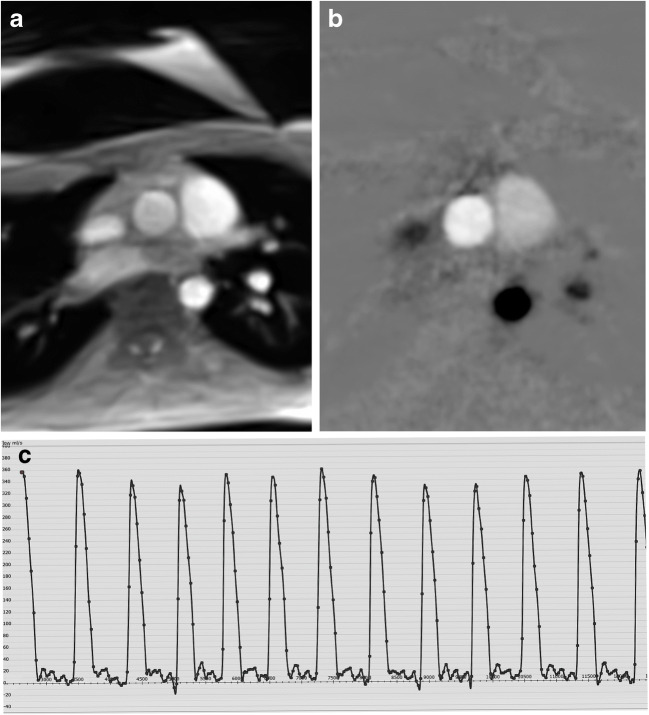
Real-time phase-contrast flow MRI of the ascending aorta in a 17-year-old male adolescent with funnel chest and vacuum bell at low pressure (same child as in Online Supplementary Material 9). a Anatomical image. b Velocity map. c Time course of evaluated flow volume. Please note the reduced phase noise in (b) from the use of a model-based reconstruction technique and the respiration-dependent variation of the flow volume in (c)
